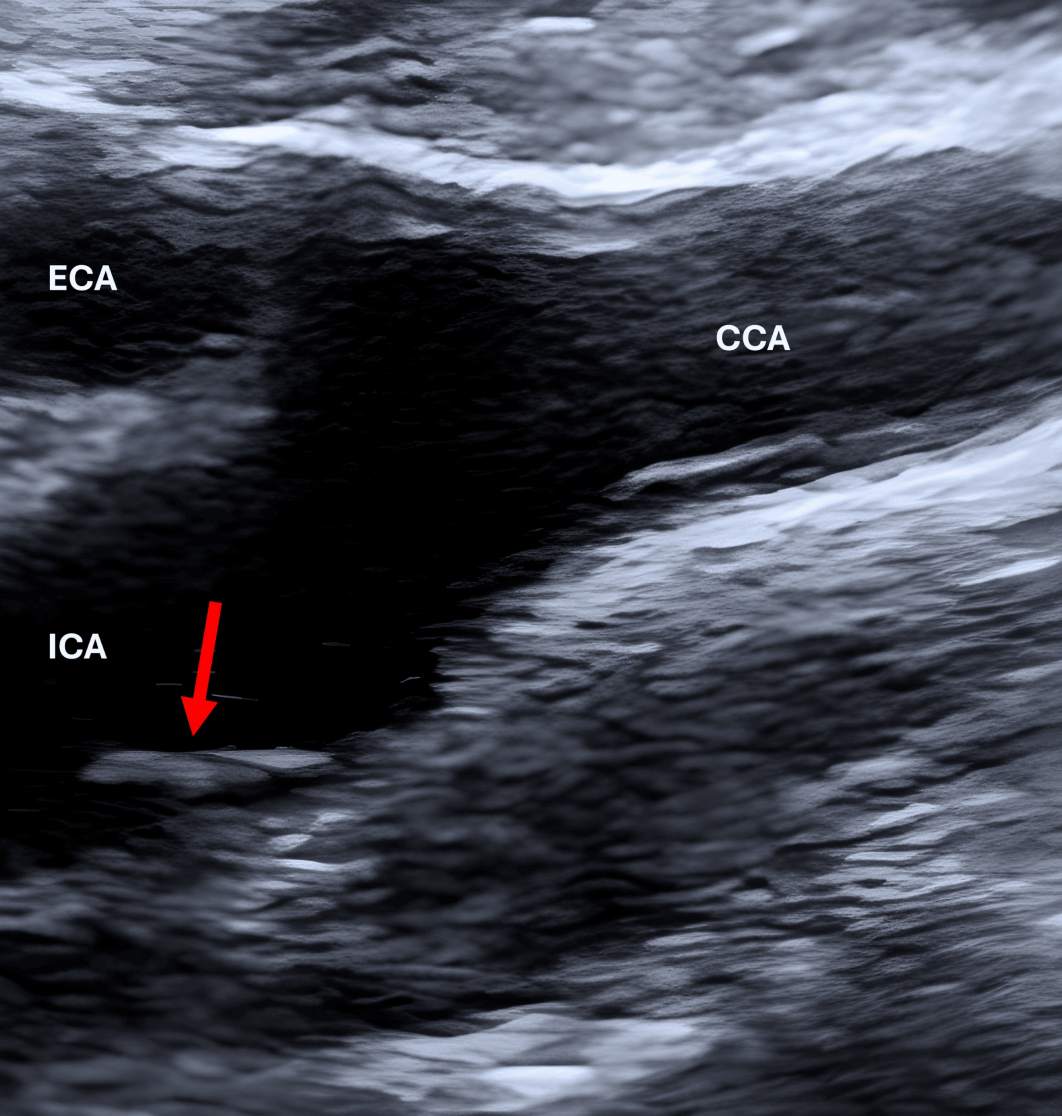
DUS showing shelf-like echogenic projection on the posterior wall of the left ICA (red arrow). DUS: Doppler ultrasound; ICA: internal carotid artery; ECA: external carotid artery; CCA: common carotid artery.
